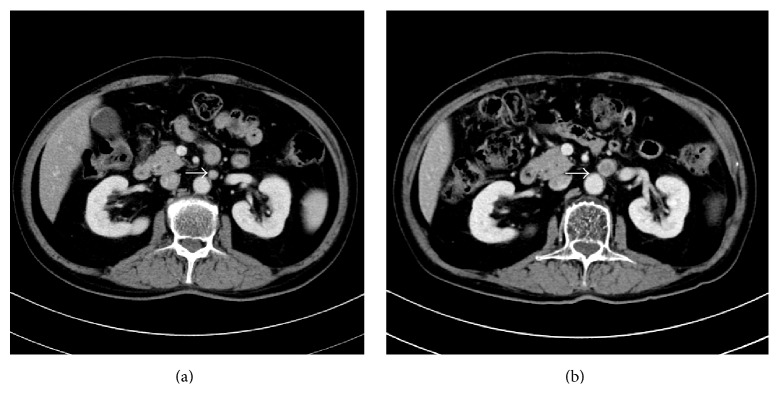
CT imaging of an enlarged para-aortic lymph node. Abdominal CT demonstrated an enlarged isolated para-aortic lymph node (a) in 2011. The lymph node was found to have enlarged to 15 mm in the minor axis (b) in 2014.
